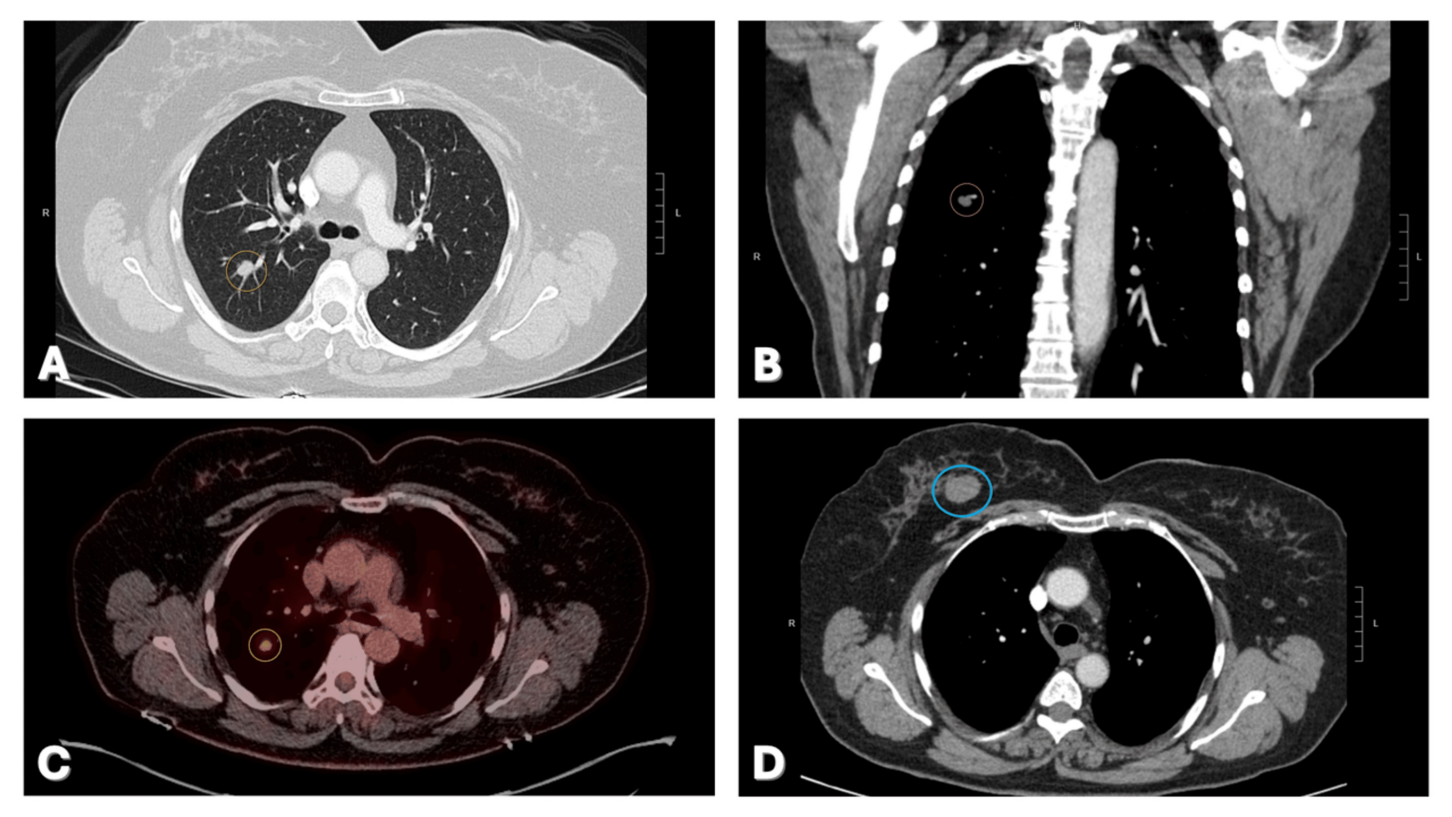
Patient's routine surveillance imaging A) Axial CT view showing the oval, 1.1 cm x 0.9 cm, right upper lobe pulmonary nodule (yellow circle). B) Coronal CT view showing the right upper lobe lung nodule (yellow circle). C) PET/ CT scan showing the hypermetabolic right upper lobe nodule (SUV of 4.6) (yellow circle). D) Axial CT view showing the 2.5 cm nodule upper central right breast (blue circle).
